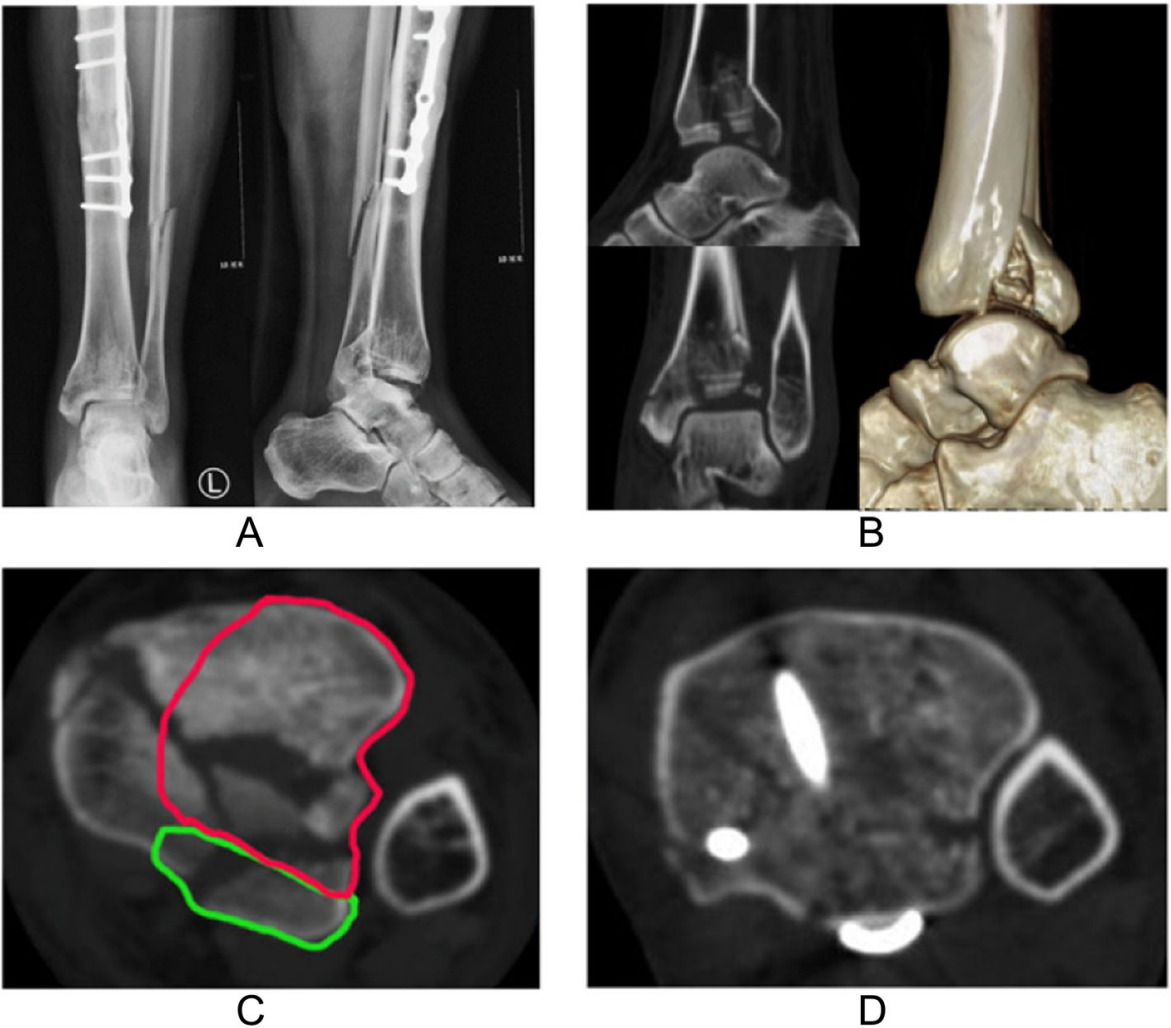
Imaging data of a 44-year-old male patient. a AP and lateral radiographs present AO/OTA 43 B2 fracture, with the ipsilateral tibia having previously undergone plate fixation. b Preoperative CT plain scan and reconstruction after removal of fibula. c Axial CT image 5 mm proximal to the tibial plafond shows comminuted fracture; red and green curve lines show areas that can be exposed intraoperatively through the anterior and posterior intervals, respectively. d CT image in the same position as c shows good reduction of the fragments and distal syndesmosis
